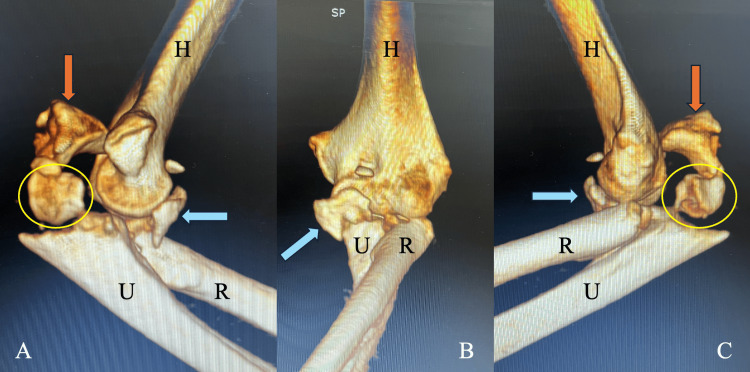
Three-dimensional computed tomography reconstructions of the left elbow demonstrating a terrible triad injury associated with an olecranon fracture. (A) Medial view showing the radial head fracture (yellow circle), coronoid fracture (blue arrow), and olecranon fracture (orange arrow). (B) Anterior view highlighting the coronoid fracture (blue arrow). (C) Lateral view demonstrating the radial head fracture (yellow circle), coronoid fracture (blue arrow) and posterior displacement of the olecranon fragment (orange arrow). H: humerus; U: ulna; R: radius.
